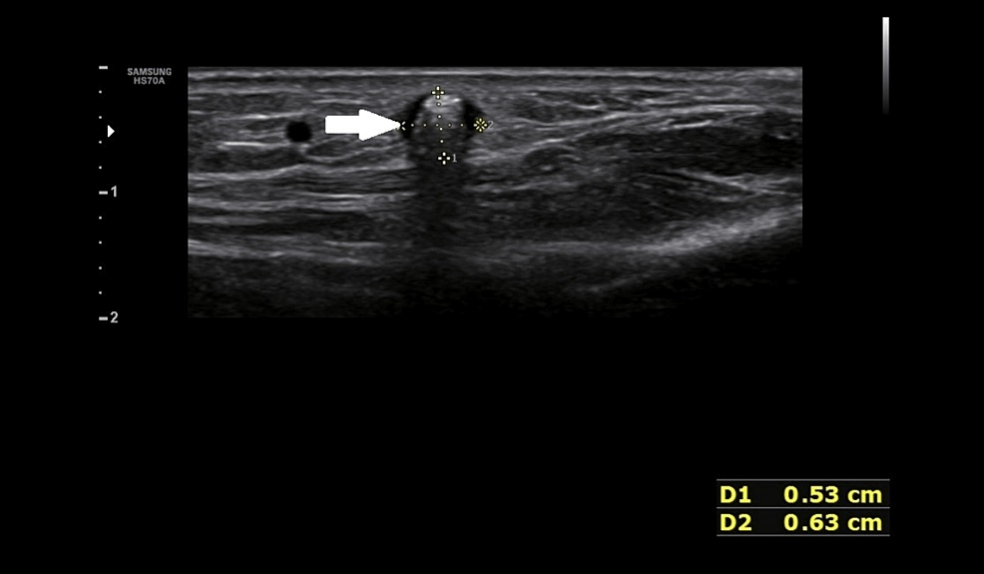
B mode ultrasonography image showing posterior acoustic shadowing (arrow)
